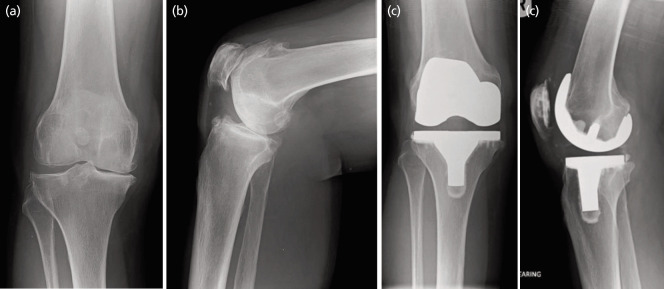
Patient 2, (a) pre-operative antero-posterior radiograph, (b) pre-operative lateral radiograph, (c) post-operative antero-posterior radiograph at one-year follow-up, and (d) post-operative lateral radiograph at one-year follow-up.
